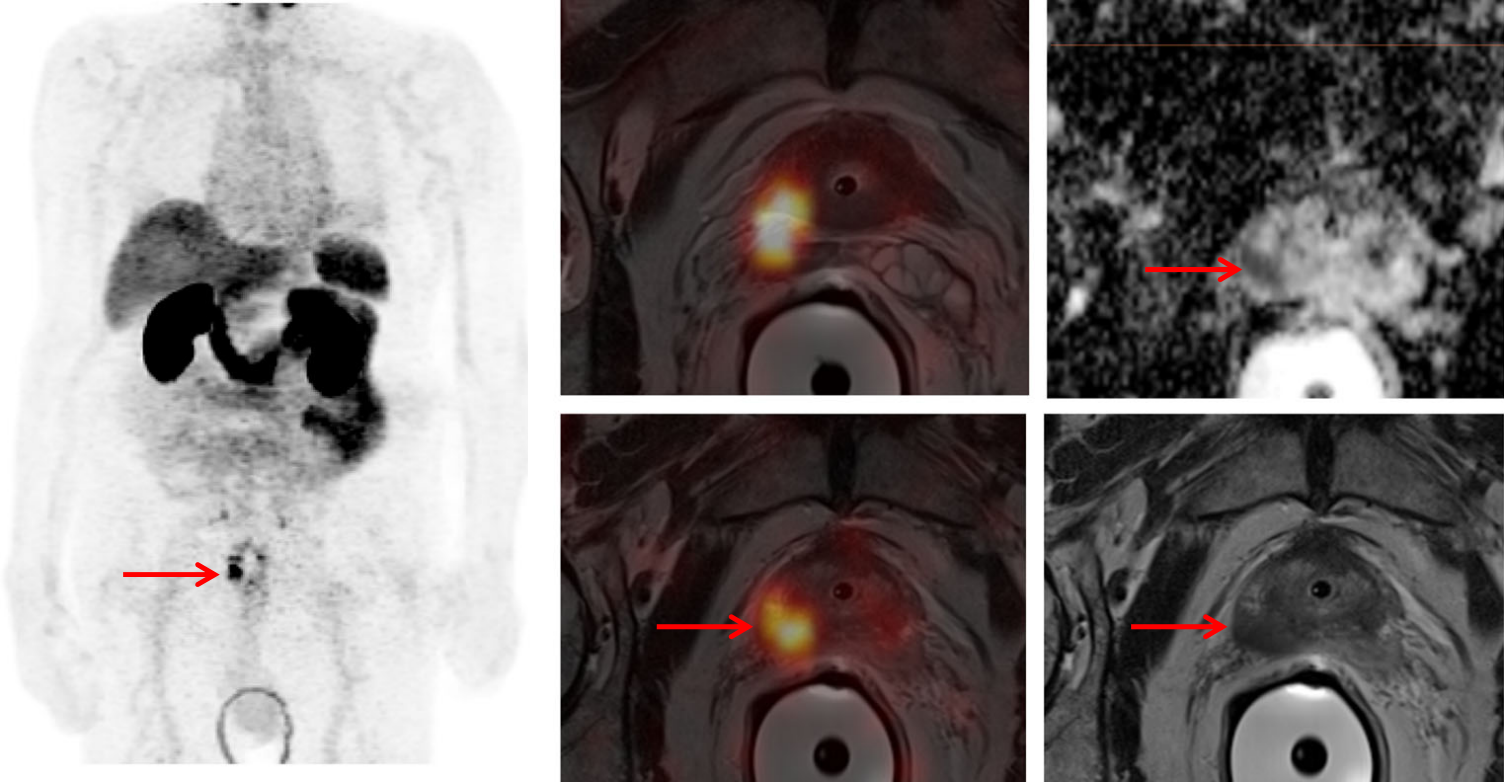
PET/MRI demonstrating the primary PC (PSA 16 ng/ml, GS 3 + 4) in the right prostate lobe (*red arrow*) invading the seminal glands with markedly increased ^68^Ga-PSMA uptake. The tumor presents with restricted diffusion on apparent diffusion coefficient (ADC) mapping and is hypointense on T2-weighted MRI
